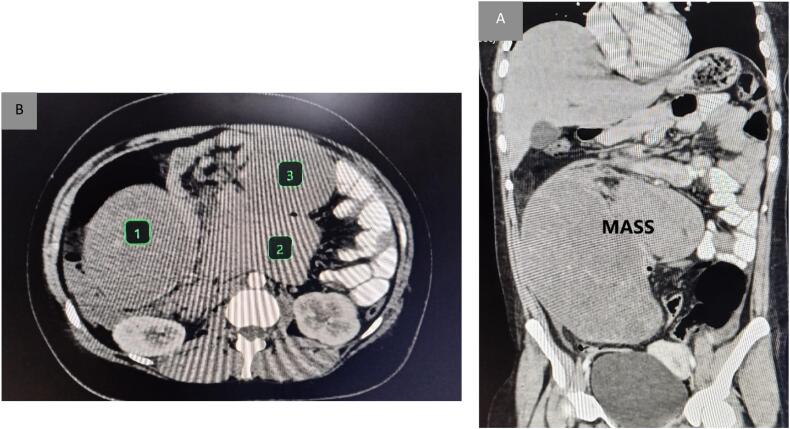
(a) Axial image from contrast-enhanced CT reveals three large connected mesenteric masses (stars) which are intimately associated with mesenteric vessels. (b) Coronal image from contrast-enhanced CT shows mildly enhancing low-attenuation mass in the abdomen (star).
